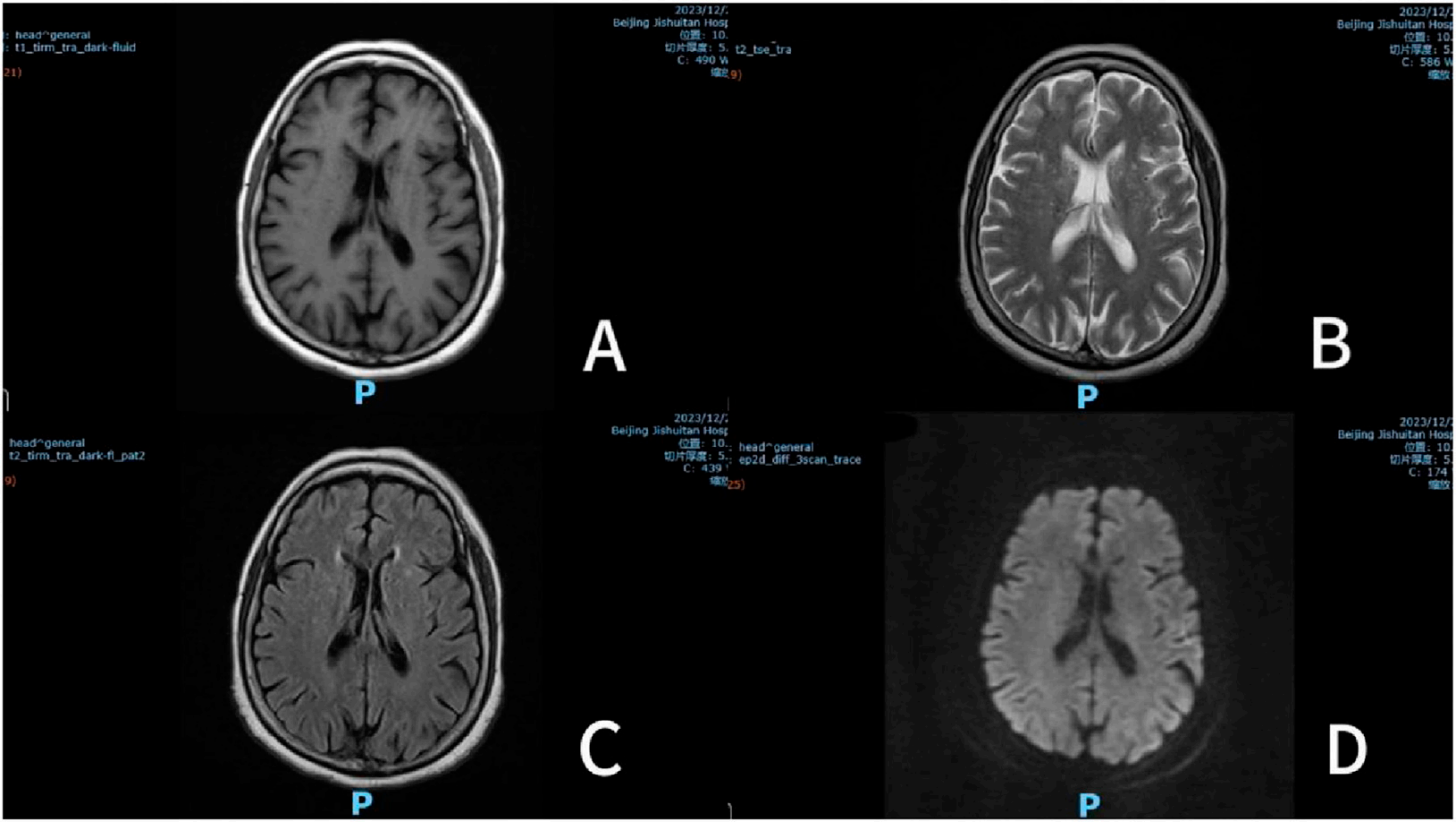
Multisequence brain MRI (December 27, 2023). Note: (A) T1 weighted imaging (T1WI); (B) T2 weighted image (T2WI); (C) Fluid attenuated inversion recovery (T2 FLAIR); (D) Diffusion-weighted imaging (DWI).
